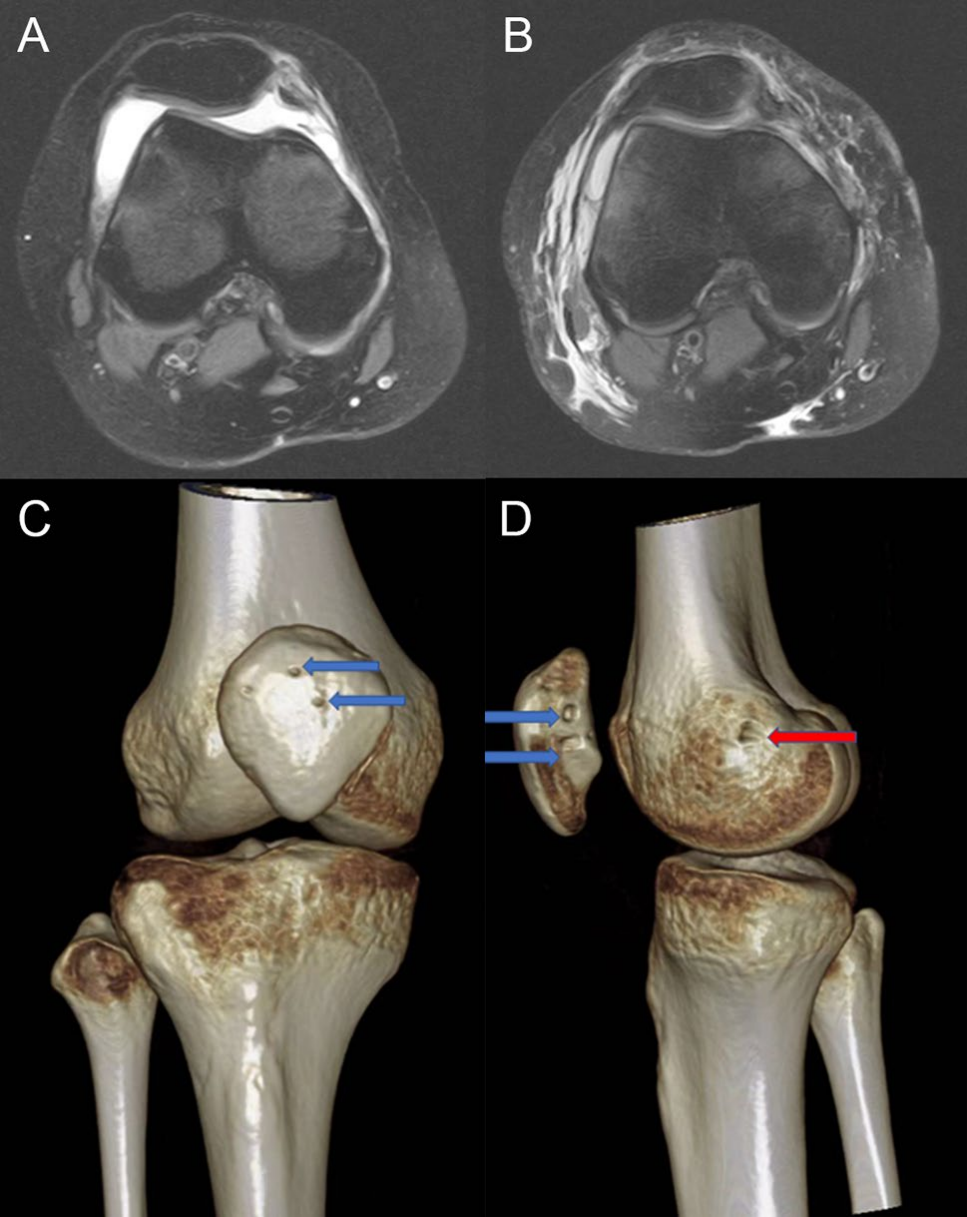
(A) Preoperative MRI showed lateral dislocation of the patella. (B) Postoperative MRI showed that the continuity of the MPFL was restored. Postoperative CT showed the location of the patellar canal (blue arrow) (C) and the external row nail (red arrow) (D)
